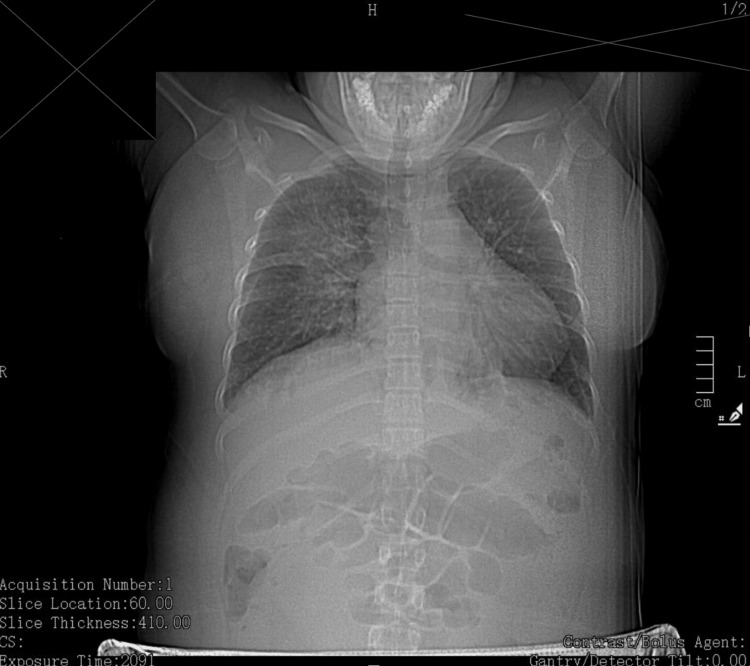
Chest CT scan showing right-sided perihilar infiltrate and bilateral pleural effusions (right > left) CT: computed tomography
